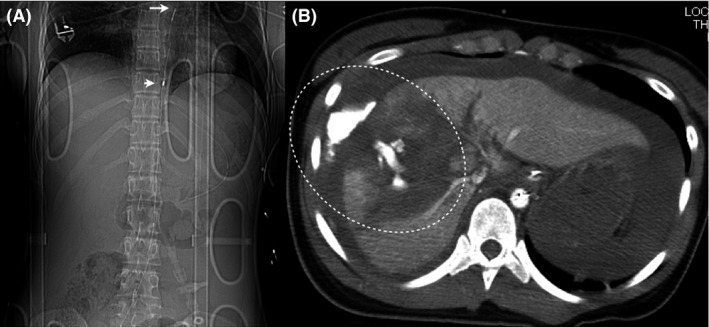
Computed tomography scout image of a 19‐year‐old woman with hemodynamic instability caused by a high‐grade hepatic injury revealed the position of the intra‐aortic balloon occlusion catheter. A, The tip of the catheter (long arrow) and proximal end of the balloon (short arrow) are shown. B, Contrast‐enhanced computed tomography (CT) revealed the extravasation of contrast medium from hepatic trauma (circle). Classified as CT grade IV according to the American Association for the Surgery of Trauma CT scale.
